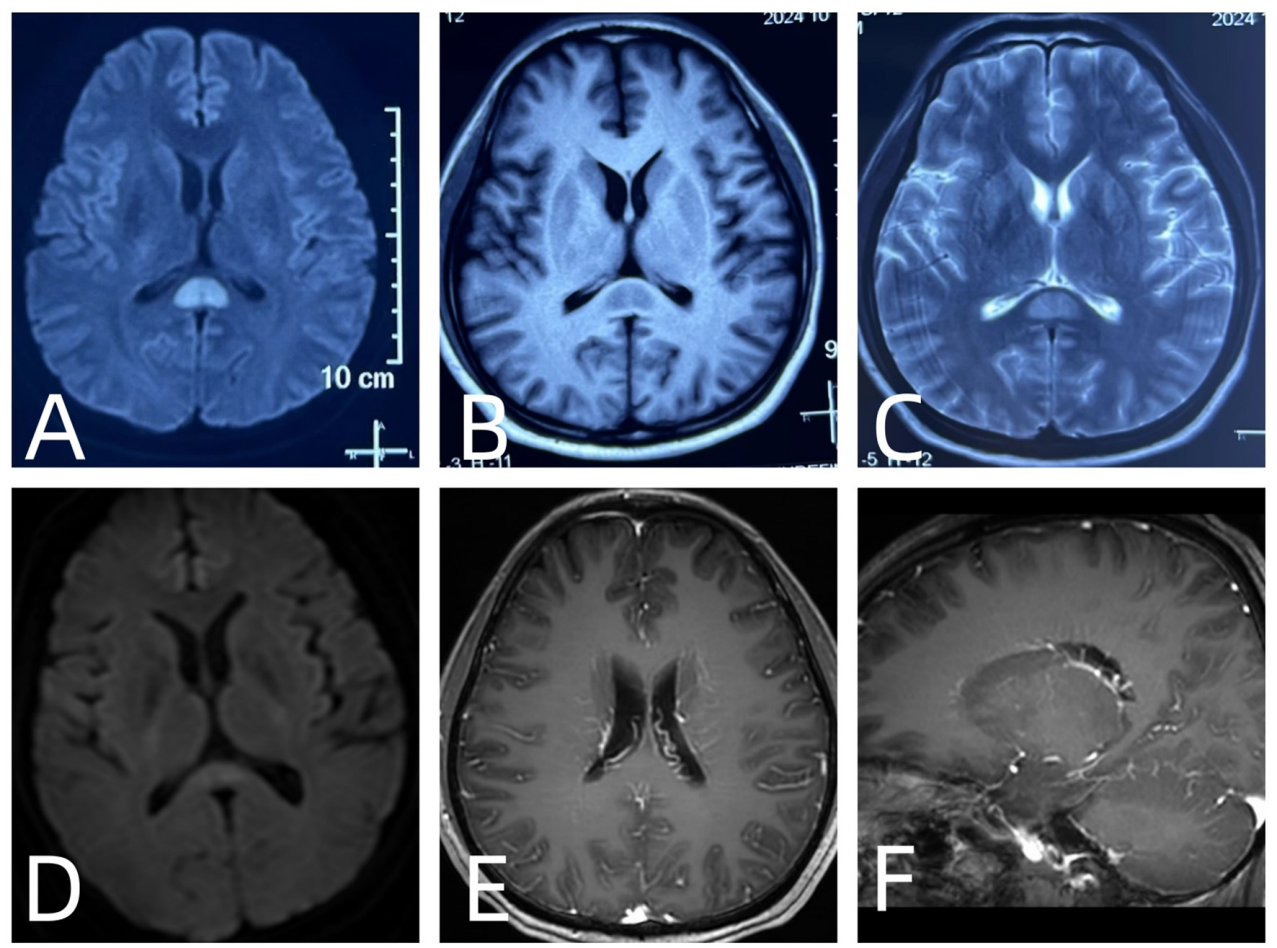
(A) diffusion-weighted imaging (DWI): hyperintense signal in the splenium of the corpus callosum. (B) T1-weighted imaging (T1WI): hypointense signal in the splenium of the corpus callosum. (C) T2-weighted imaging (T2WI): hyperintense signal in the splenium of the corpus callosum. (D) Follow-up DWI: resolution of the previously observed hyperintense signal in the splenium of the corpus callosum. (E, F) Post-contrast imaging: linear perivascular enhancement radiating outward from the lateral ventricles.
